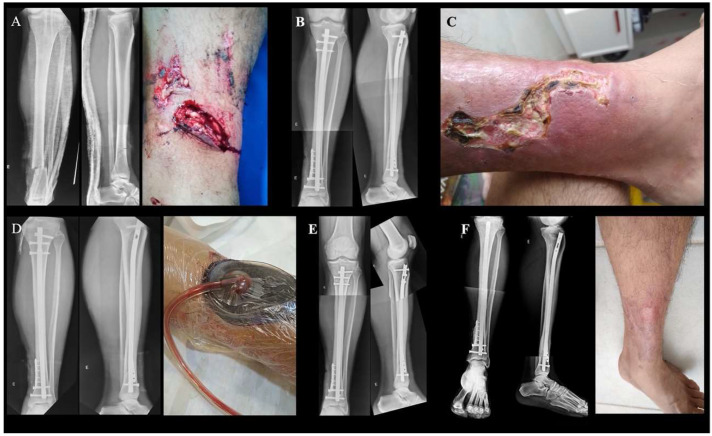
(A) 53-year-old man was involved in a motorcycle accident and sustained an open fracture on the left tibia. The patient reported having insulin-dependent diabetes. (A) AP and lateral radiographs showed a simple fracture of the distal tibia, but the degree of soft tissue involvement and severe contamination led the injury to be classified as Gustilo et al. grade 3A. (B) The patient was taken to the operating room (OR) for urgent irrigation and debridement, and immediate definitive fixation with a medial mini-fragment reduction plate and a reamed tibial intramedullary nail (IM). (C) Ten days after hospital discharge, the patient returned to the outpatient clinic complaining of pain in the operated leg and showing clear signs of acute FRI. (D) The patient was re-admitted to the hospital and taken to the operating room for wound irrigation and debridement. Samples were collected for culture. Due to the acute nature of the FRI, it was decided to initiate appropriate suppressive antibiotic therapy and maintain the IM implant. A negative pressure dressing was placed. (E) AP and lateral radiographs of the left leg taken 90 days after the second procedure showed uneventful healing of the fracture. (F) AP and lateral radiographs of the left leg after 2 years of follow-up showed no signs of infection and adequate healing of the soft tissues.
